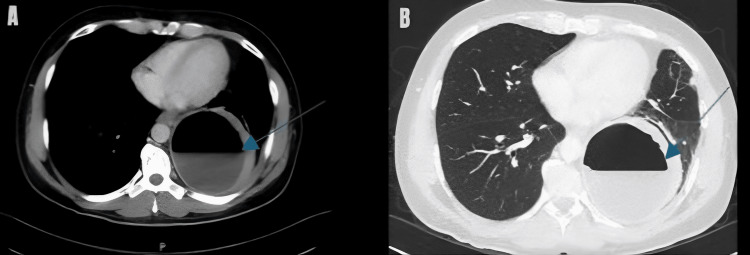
Contrast-enhanced CT thorax. Axial (A) and coronal (B) contrast-enhanced CT images of the chest definitively characterize the left lower lobe abscess. The images demonstrate a large (9.3 × 9.6 cm), thick-walled cavitary lesion with peripheral enhancement (arrows), confirming the diagnosis of a primary lung abscess and excluding pleural involvement.
